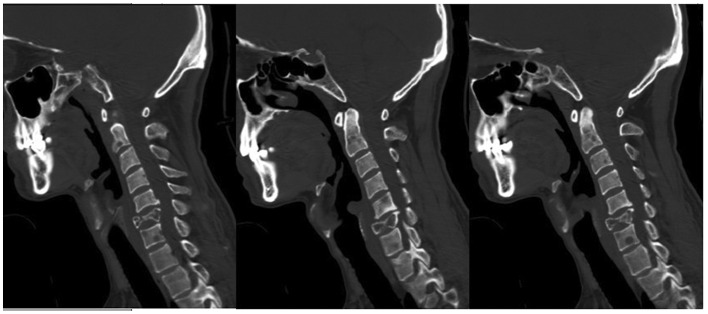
Cervical sagittal CT scans revealing complete osteolytic destruction of the C6 vertebral body and small foci in C2, C3, C4, C5, C7, T1 and T2. CT, computed tomography.
